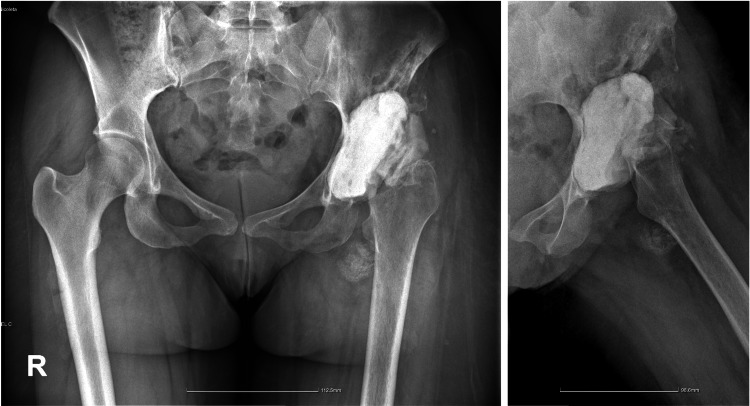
Postoperative X-ray: first surgical step This is an example of the postoperative X-ray aspect after the surgical step of debridement with the polymethyl-methacrylate mixed with an antibiotic-spacer in place (Case 1).
